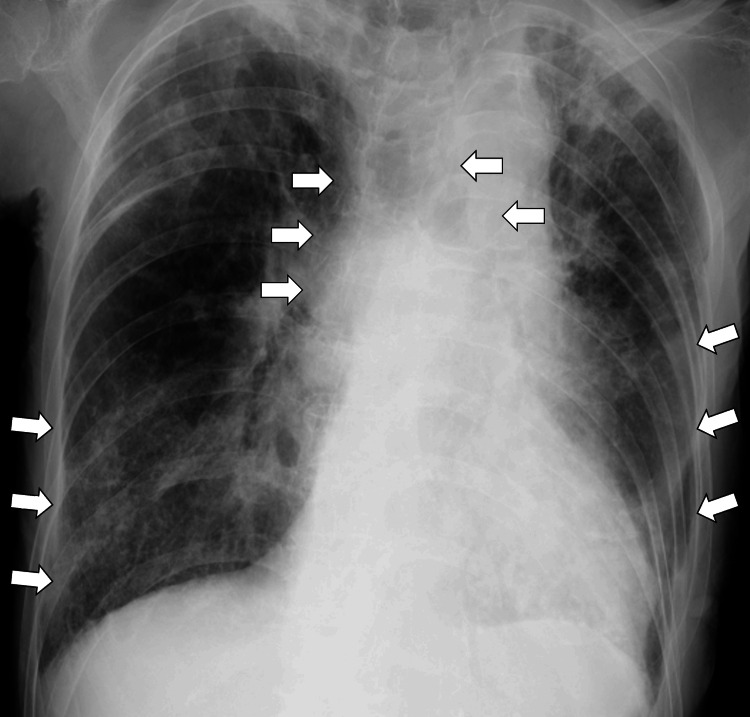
A chest X-ray showing decreased transparency in bilateral lungs and tracheal deviation to the left (white arrows)
